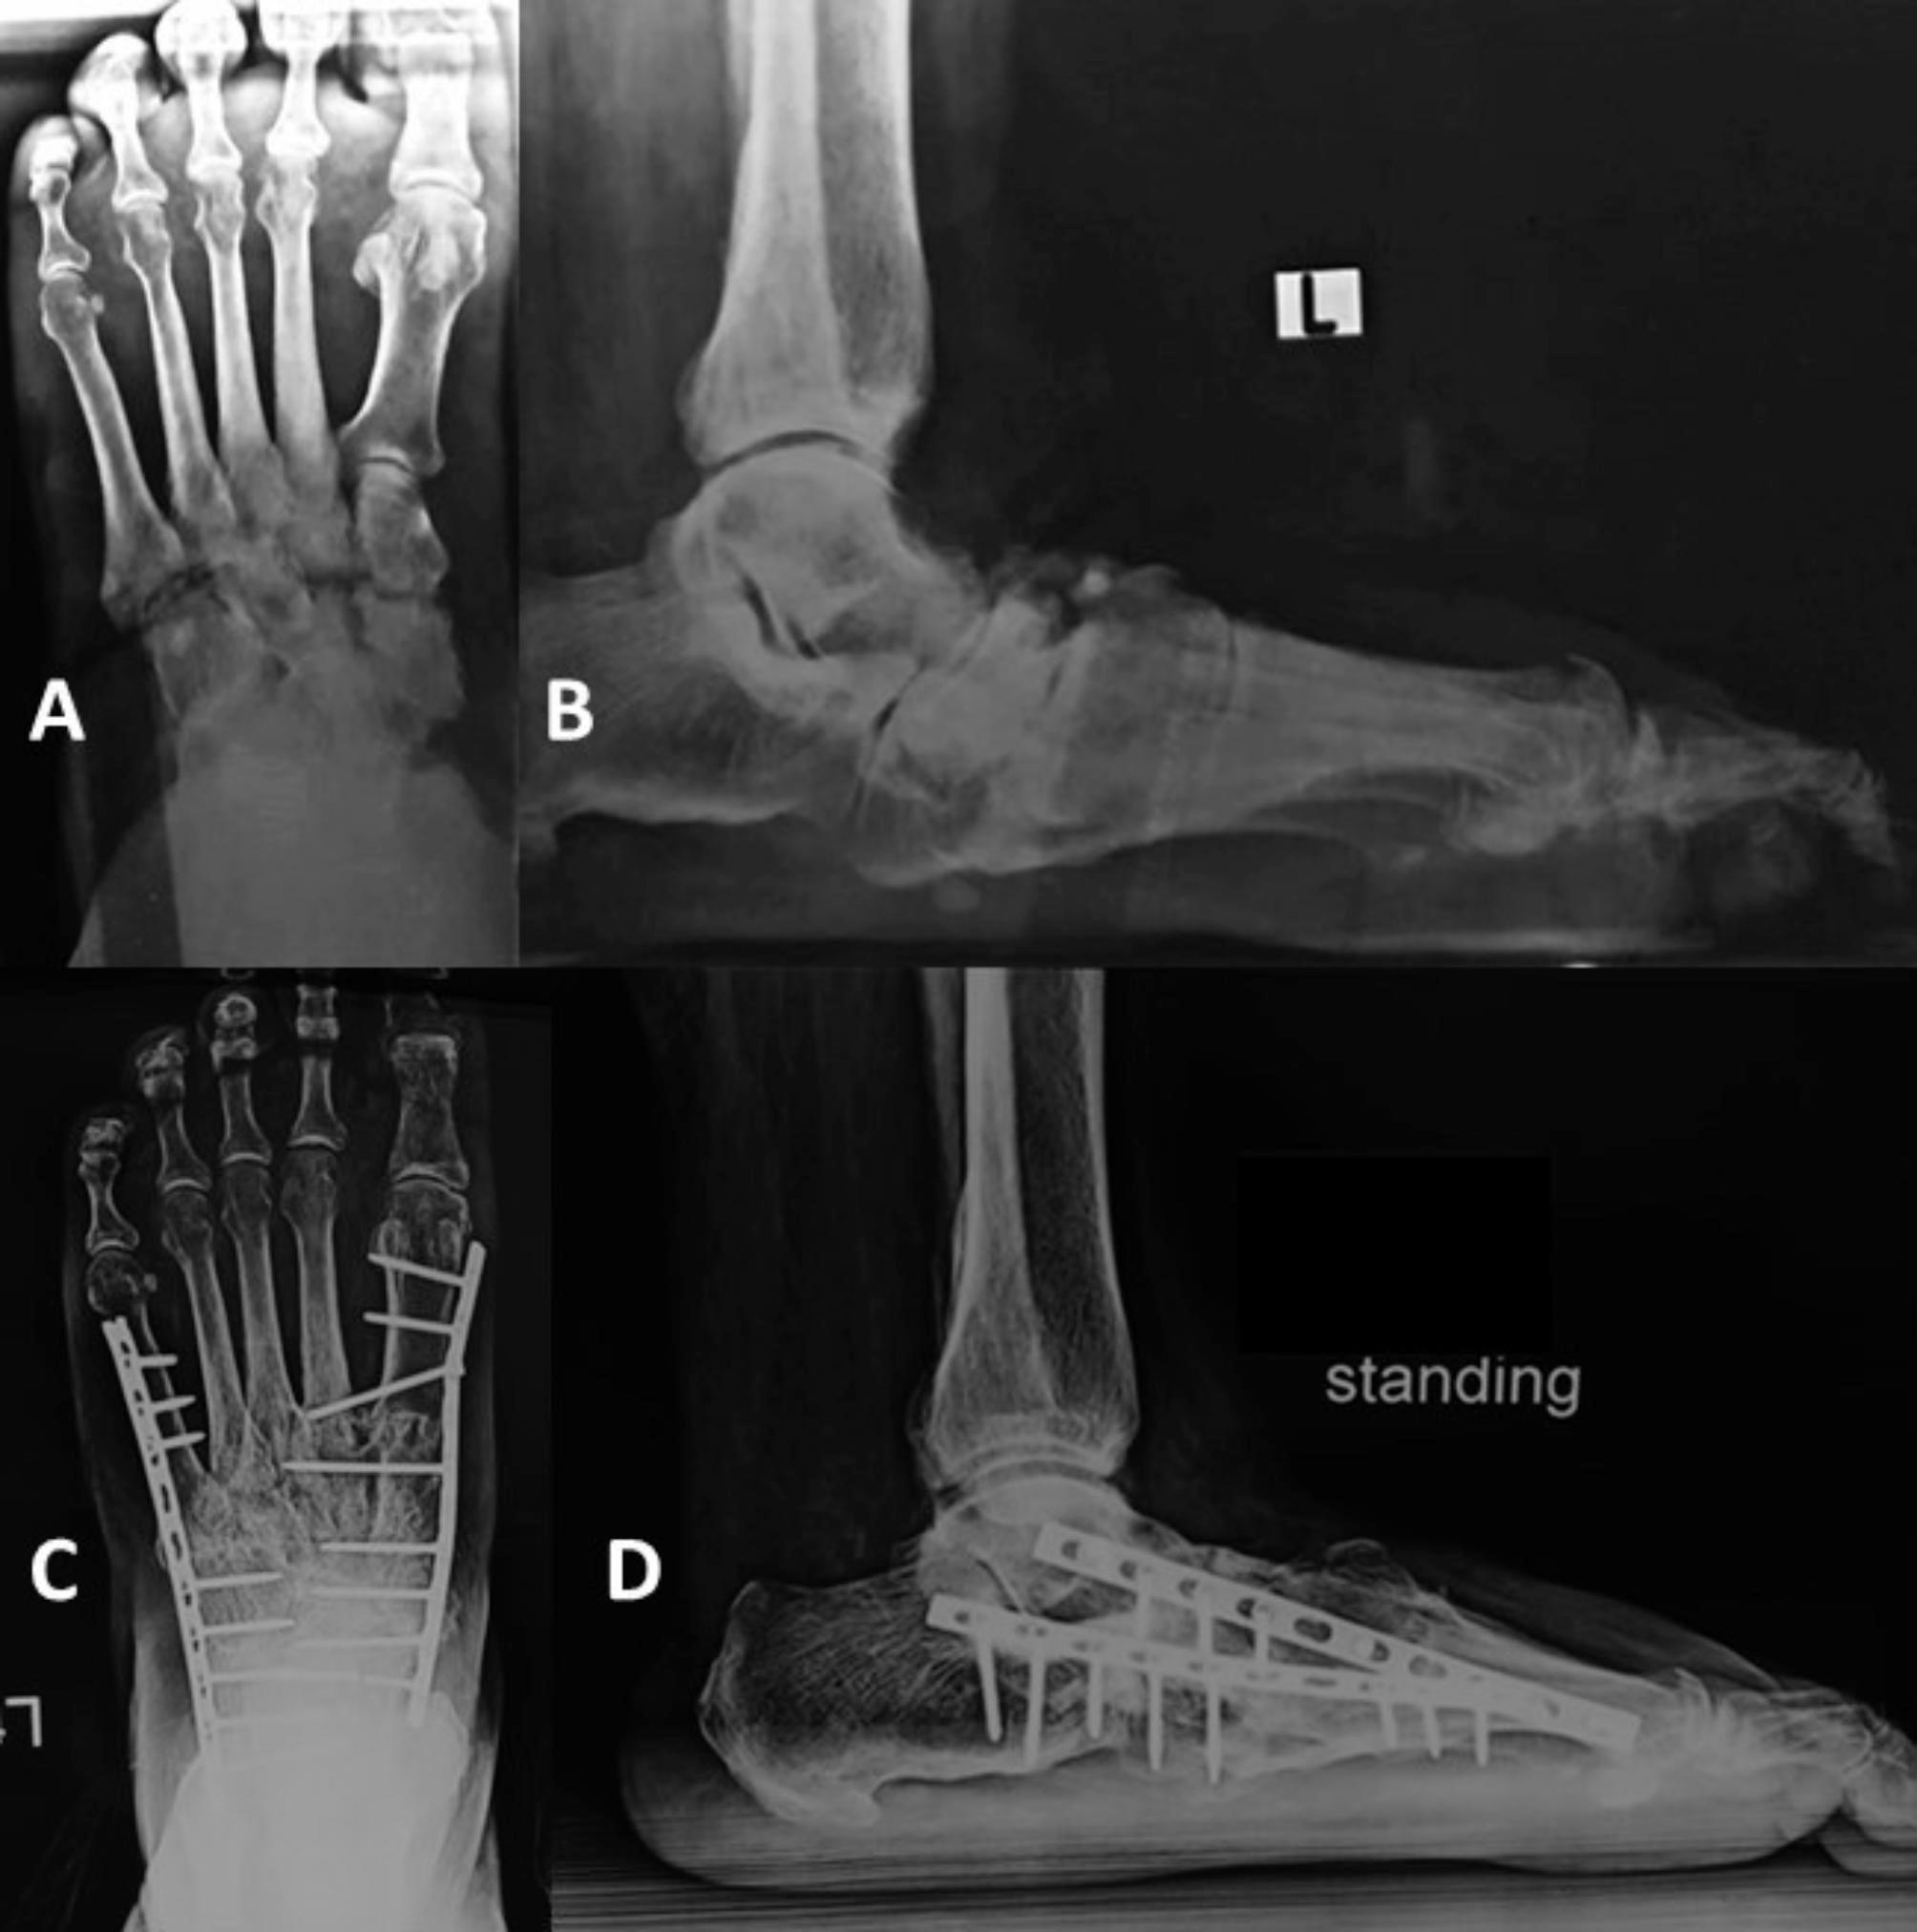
Female patient, 56 years old, diagnosed with midfoot CN with midfoot breakage. A, and B, preoperative anteroposterior and lateral plain radiographs. C and D, after 28 months of having a bi-columnar arthrodesis using plates and screws, there is a solid union of the arthrodesis sites
